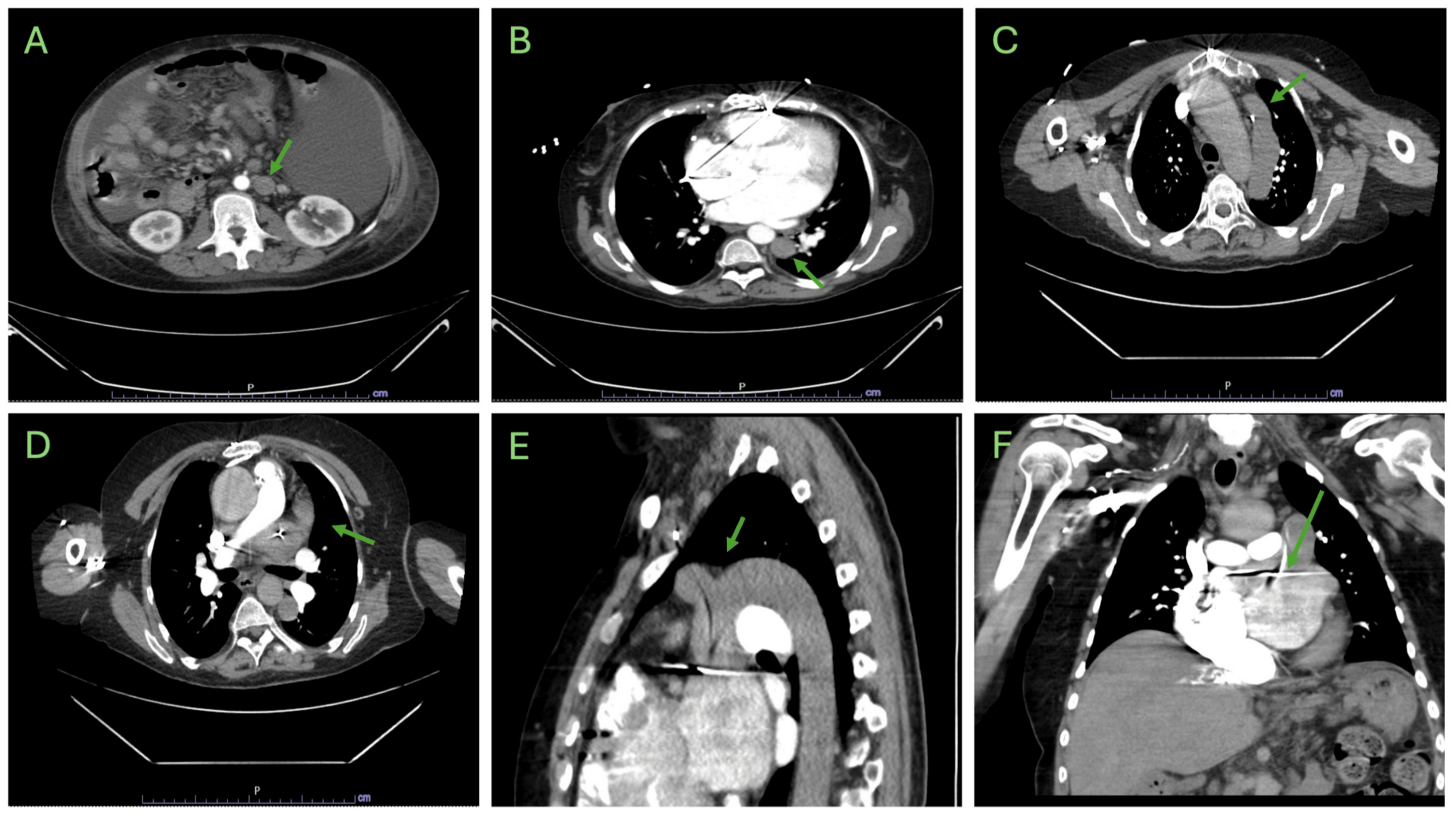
IVC is seen located slightly left and posterior to the aorta on contrast CT (A), continuing into the thorax along the hemiazygos course (B). Left-sided SVC is seen adjacent to the aorta (C), meeting up with the IVC and coursing directly into the right atrium (D). The IVC is seen connecting with the left SVC through an azygos arch (E) and draining into the right atrium through an unknown tract, highlighted by the path of the pacemaker lead (F). IVC: inferior vena cava, CT: computed tomography, SVC: superior vena cava
